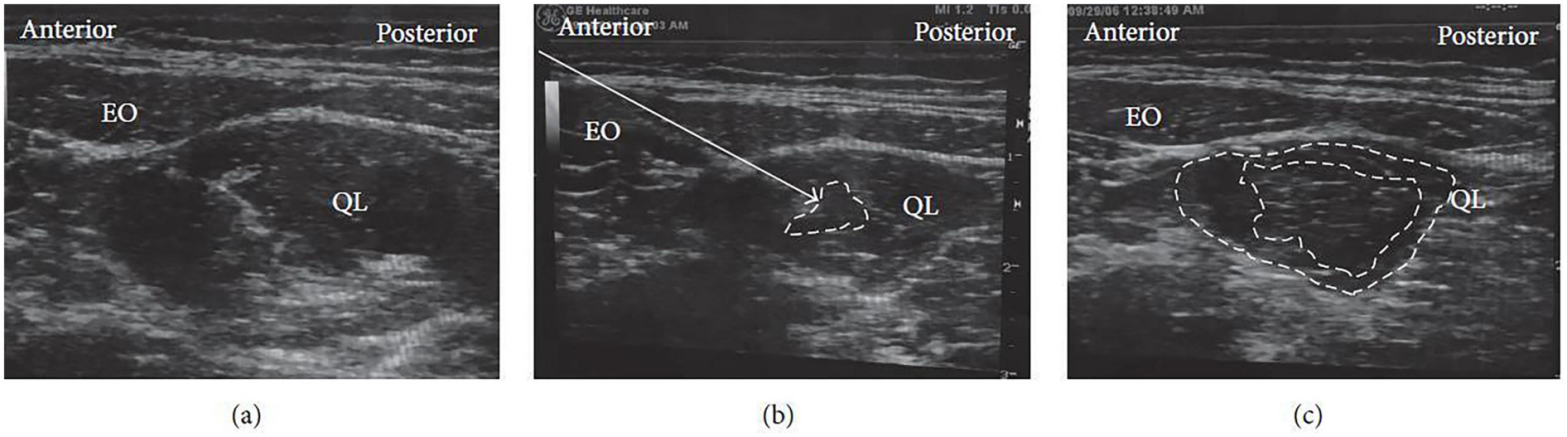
Ultrasound images of intramuscular QLB: (a) before injection, (b) during test injection, and (c) after injection. EO, external oblique; QL, quadratus lumborum. White arrow: needle trajectory; white dotted line: spread of local anesthetic within (b) or between (c) muscle layers [Reproduced from Ueshima et al. (28). © John Wiley & Sons, Inc. Distributed under the Creative Commons Attribution License, CC BY 4.0].
